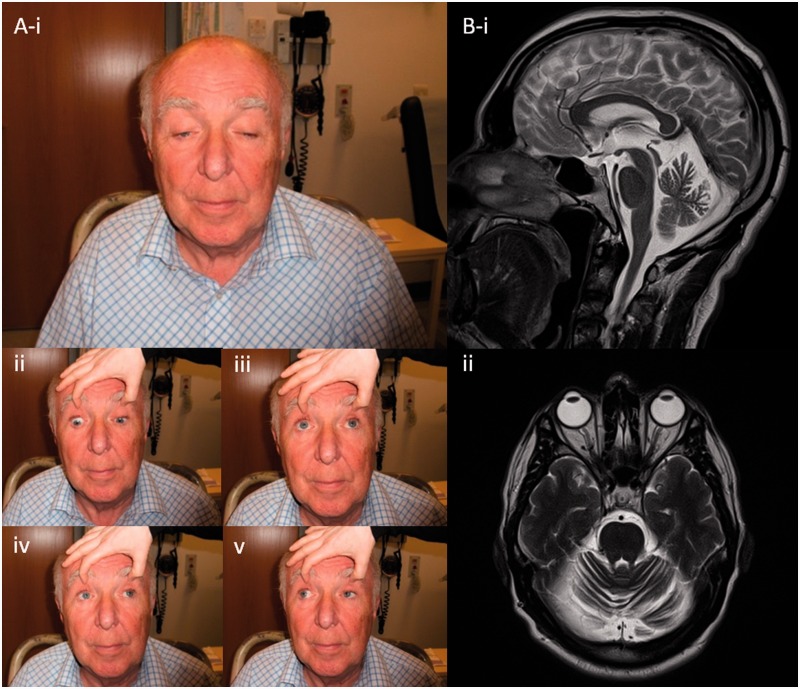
Clinical features. (A) Typical ophthalmological features of a patient with hereditary spastic paraplegia type 7 with PEO: marked ptosis is evident in primary gaze (i); extraocular motility in cardinal directions of gaze is mildly reduced, with restriction of upgaze most affected as the patient is asked to look down (ii); up (iii); left (iv); and right (v). (B) T_2_-weighted MRI images in demonstrating diffuse cerebellar volume loss, in sagittal (i); and transverse-axial (ii) planes.
